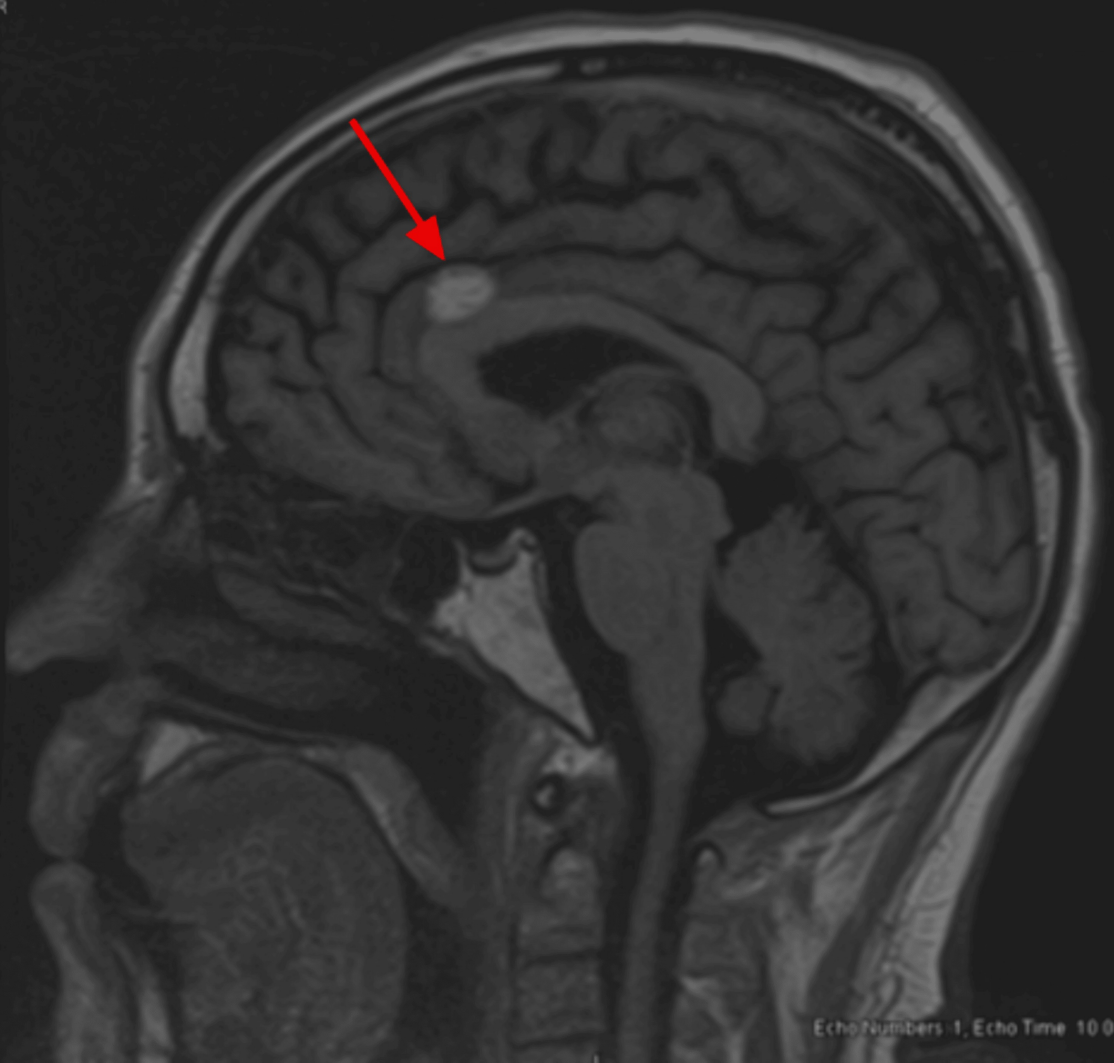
Brain MRI (May 2024) showing stability of metastases
